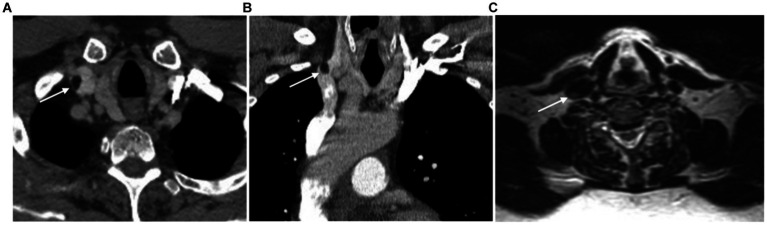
Axial (A) and coronal (B) images of a CT scan of the chest, revealing a 10 mm, well-defined, hypodense mass at the right jugulosubclavian venous confluence (white arrows). This lesion is consistent with the characteristics of an intravascular lipoma, exhibiting fat attenuation (-97 HU) and no evidence of significant contrast enhancement. On an axial T2-weighted MRI sequence without fat saturation (C), the lipoma appears as a hyperintense, homogeneous mass.
